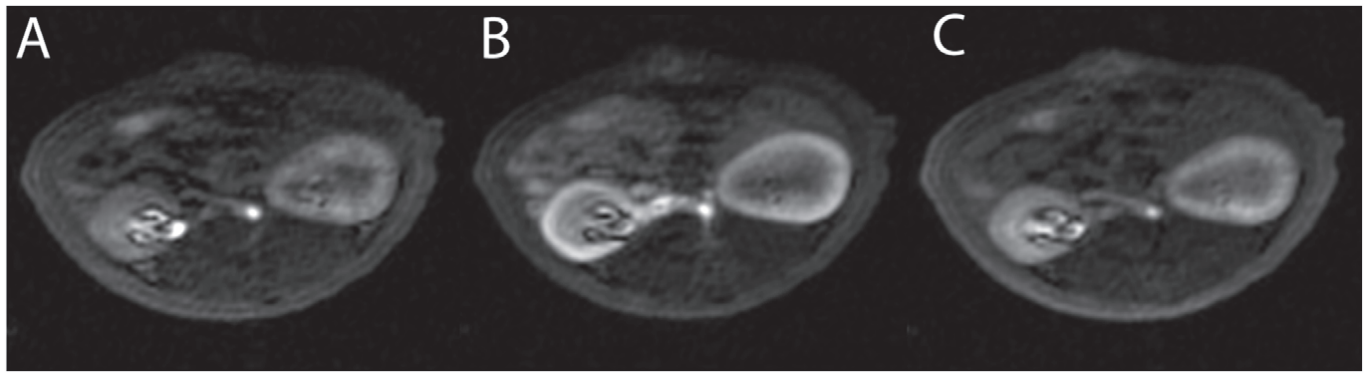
Representative DCE-MRI images. Representative DCE-MRI TWIST images at different points in time. (A) Baseline image before contrast agent injection. (B) Cortical peak contrast image approximately 40 s after bolus injection and an image (C) taken approximately 4 min later showing increased medullar contrast. Regarding the contrast uptake and its distribution, the AKI kidney can clearly be distinguished from the healthy kidney.
